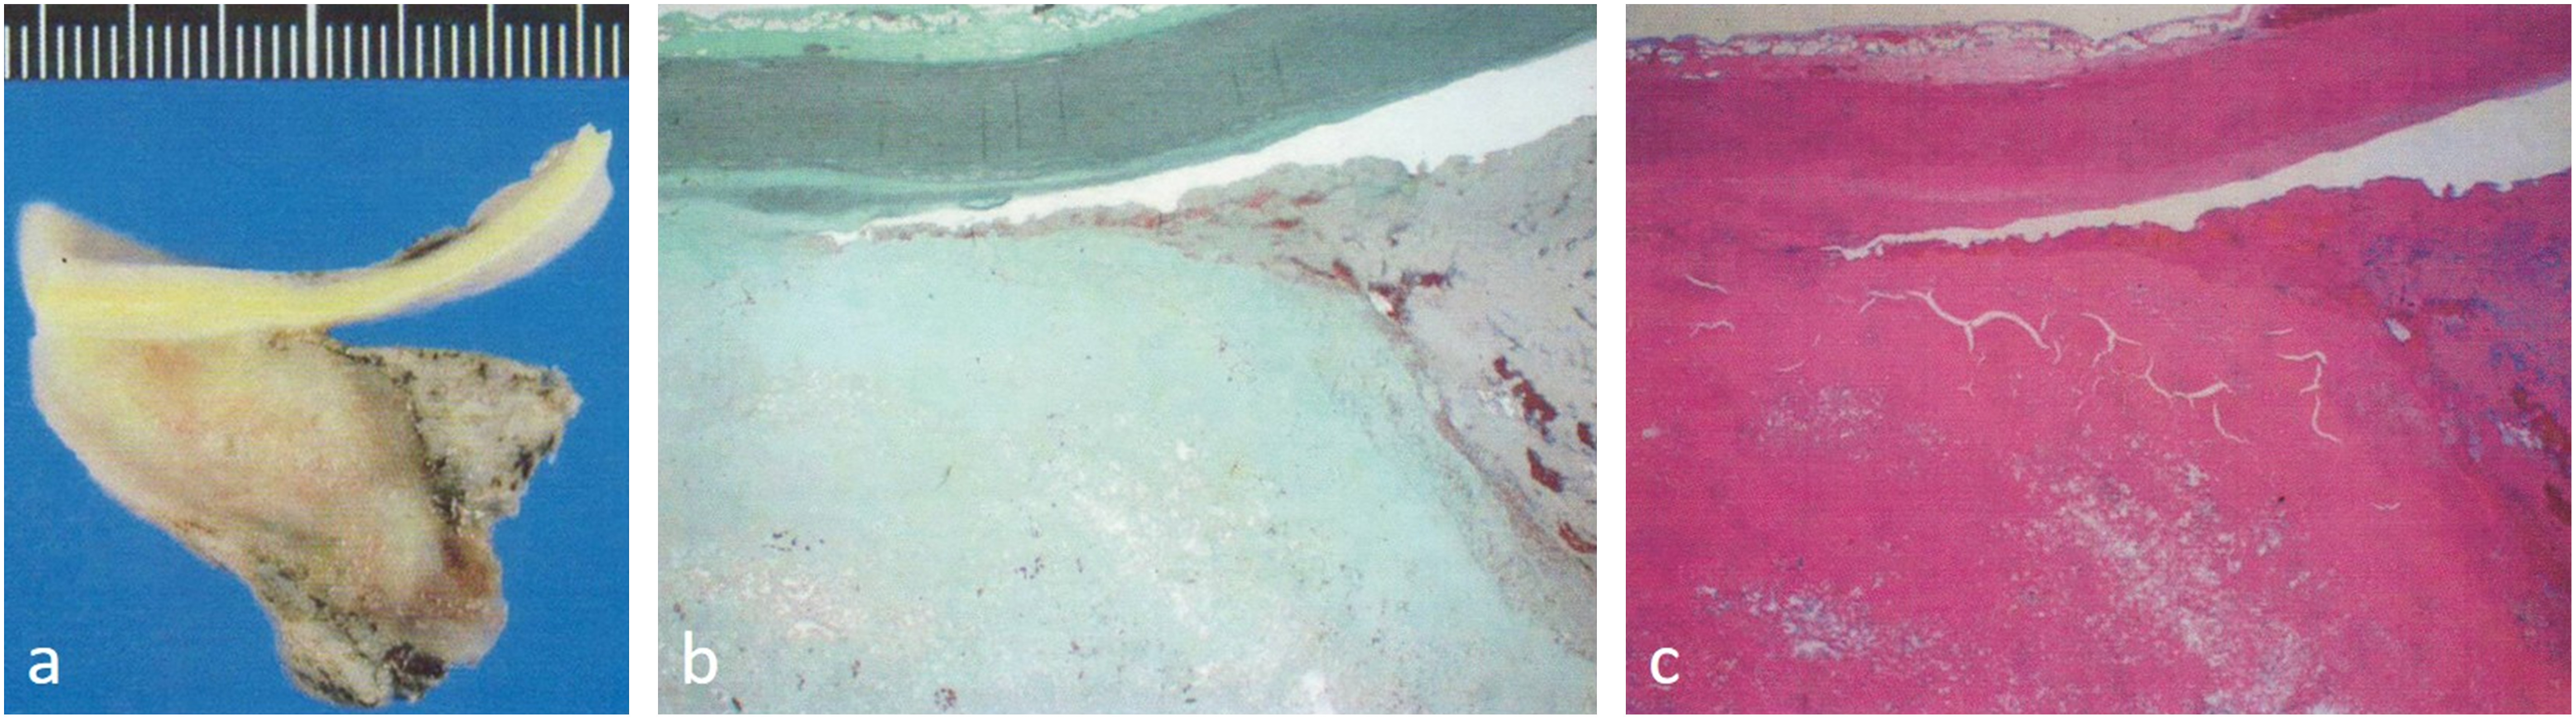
Fig. 3 (A) A contrast computed tomography showed thrombus in ascending aorta (arrow). (B) In operative findings, protruding thrombosis (16×10 mm: double arrows) in ascending aorta.
